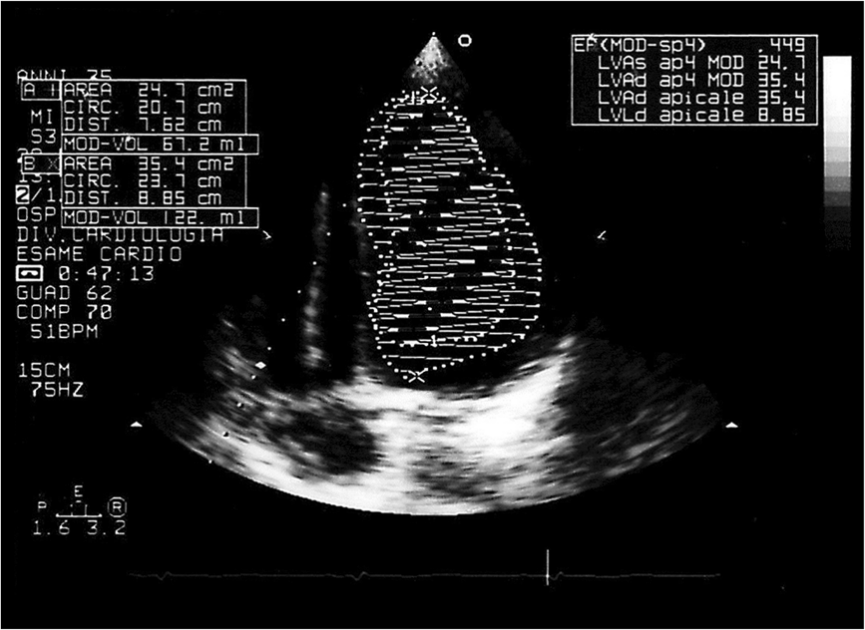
Echocardiogram showing LVEF (45%) at 1 month follow up after ivabradine introduction.
